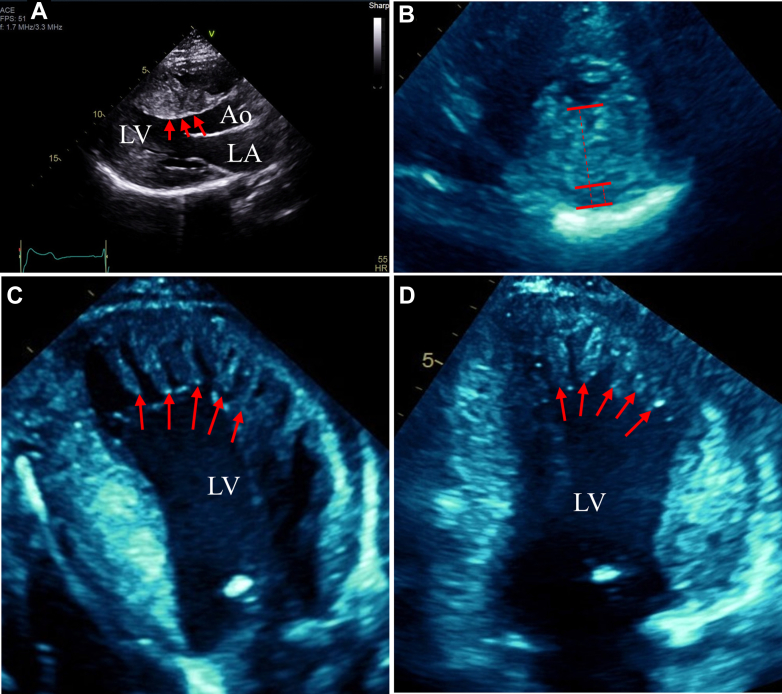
Noncompaction Pathology After Septal Myectomy The patient's echocardiographic images at age 21, nearly 2 years after septal myomectomy. The parasternal long-axis view in diastole demonstrates septal myomectomy results (red arrows) with significant reduction in septal wall thickness (A). The parasternal short-axis in end-systole (B) reflects a ratio of noncompacted to compacted myocardial thickness of >2:1, with noncompacted apical lateral and anteroapical myocardium, meeting much of the primary morphologic Jenni criteria for noncompaction.^1^ Apical 4- (C) and 2-chamber (D) views demonstrate prominent noncompacted muscle (red arrows) with large intertrabecular recesses. Ao = aorta; LA = left atrium; LV = left ventricle.
